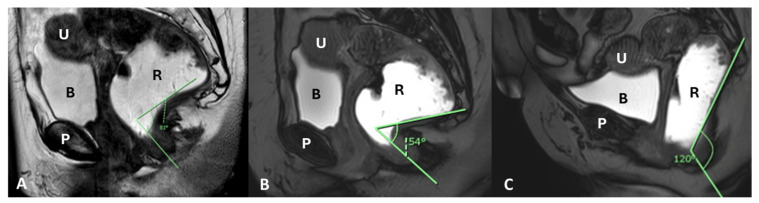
Sagittal T2-weighted MR defecography images demonstrate anorectal angle dynamics. (A) At rest post-rectal gel filling, the angle measures 81°, with the anorectal junction at the pubococcygeal line (PCL). (B) During pre-defecation contraction, the angle narrows to 54°. (C) During defecation straining, rectal prolapse widens the angle to 120°, with a 6 cm anorectal junction descent. Identifiable structures include the pubic symphysis (P), rectum (R), bladder (B), and uterus (U).
